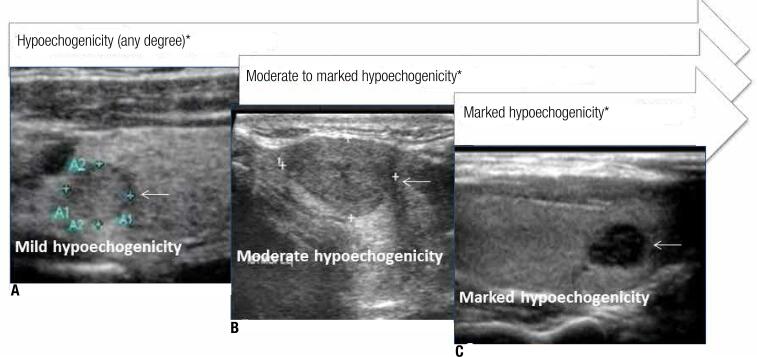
Hypoechogenicity gradation in thyroid nodules. Ultrasound images exemplify nodules that exhibit three grades of hypoechogenicity: (arrows). A. Mild hypoechogenicity: nodule presents echogenicity lesser than thyroid parenchyma and greater than the strap muscle (arrow). B. Moderate hypoechogenicity: nodule presents echogenicity similar to the strap muscle (arrow). C. Marked hypoechogenicity: nodule presents echogenicity lesser than the strap muscle (arrow). * Included in bivariate and multivariate analysis.
